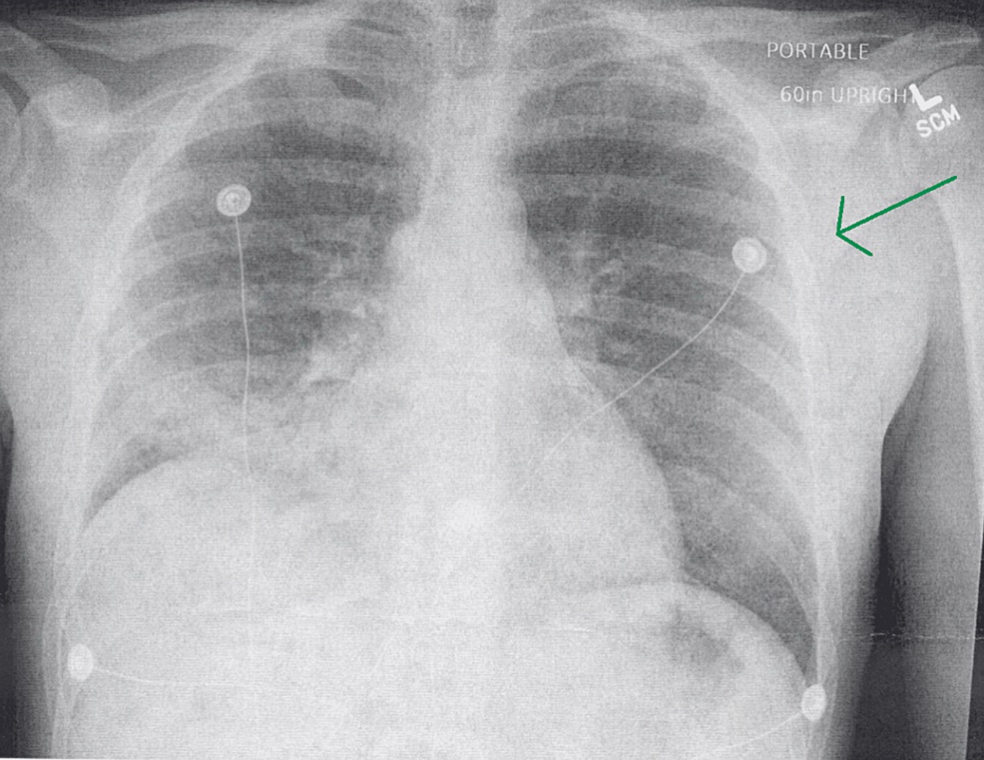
Anterior-posterior chest X-ray from initial visit
